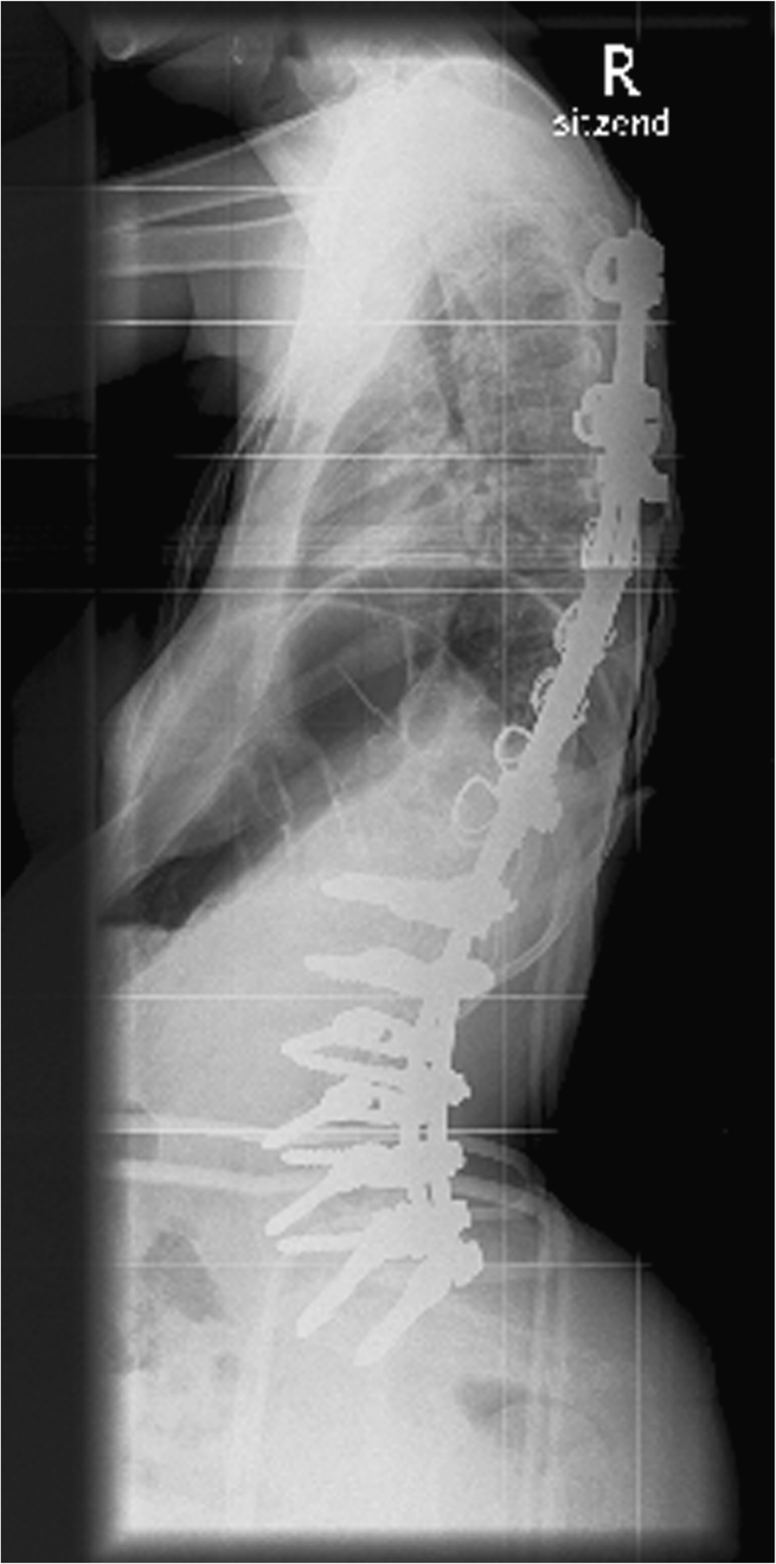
Lateral X-ray of the sp ine at follow-up: Cobb angle of 46° (Th7-L2-L5) left-convex , 30° pelvic tilt to the left.
